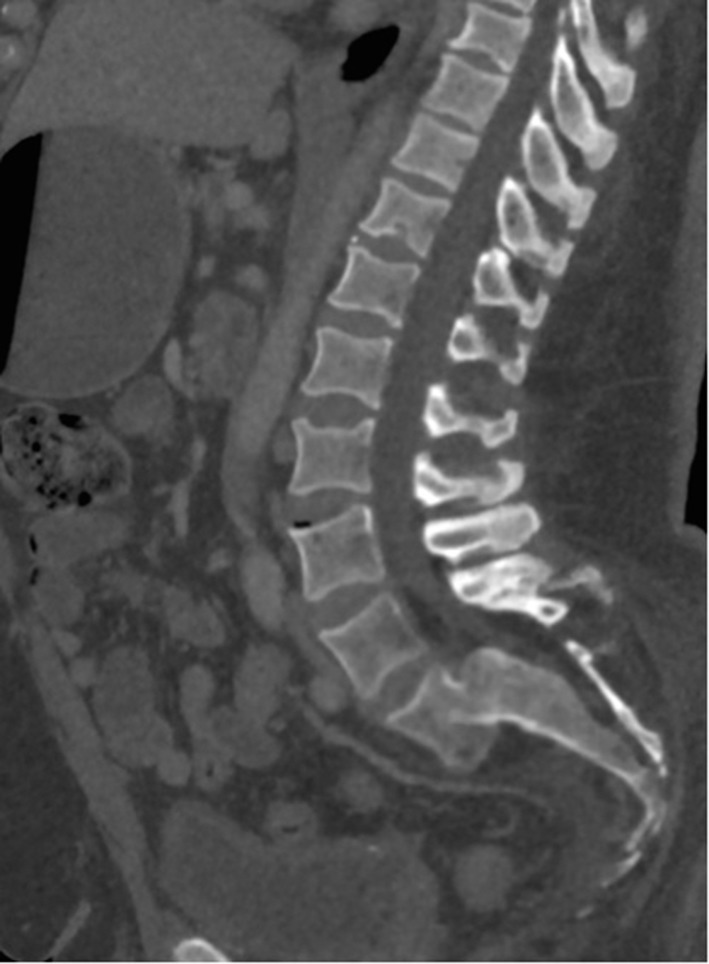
Midline sagittal reconstruction CT demonstrating Grade III degenerative spondylolisthesis of the L5 vertebral body on the S1 vertebral body with evidence of spontaneous arthrodesis.
